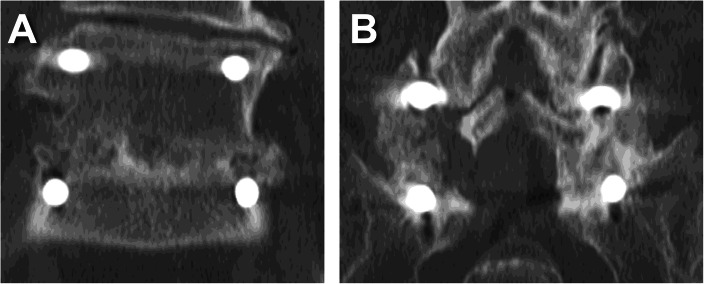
Representative coronal CT scans of a male patient in his 60s at two years postoperative showing A bridging callous across the L5-S1 interbody fusion and B posterolateral fusion mass (Lenke B, probably solid with a unilateral stout fusion mass and a contralateral thin fusion mass)
